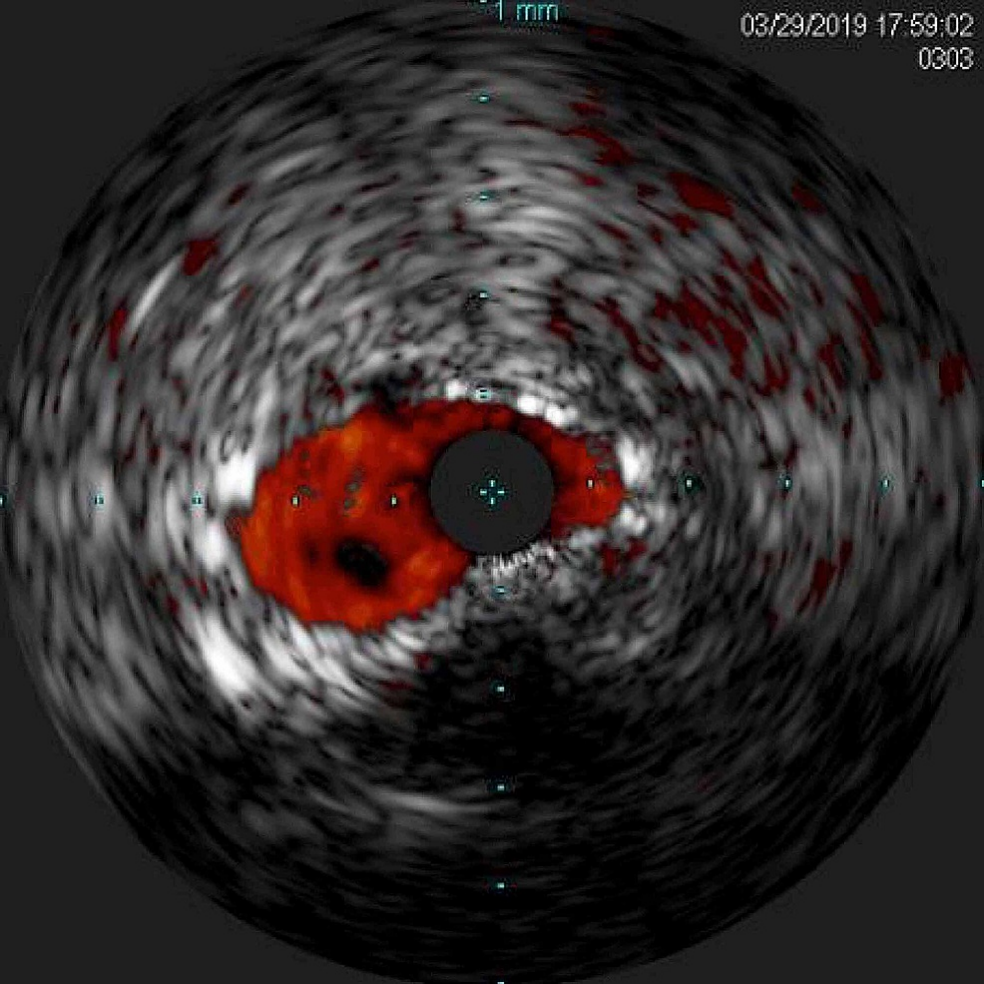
Intravascular ultrasound demonstrating an under-expanded stent in the distal LMCA. LMCA: left main coronary artery.
